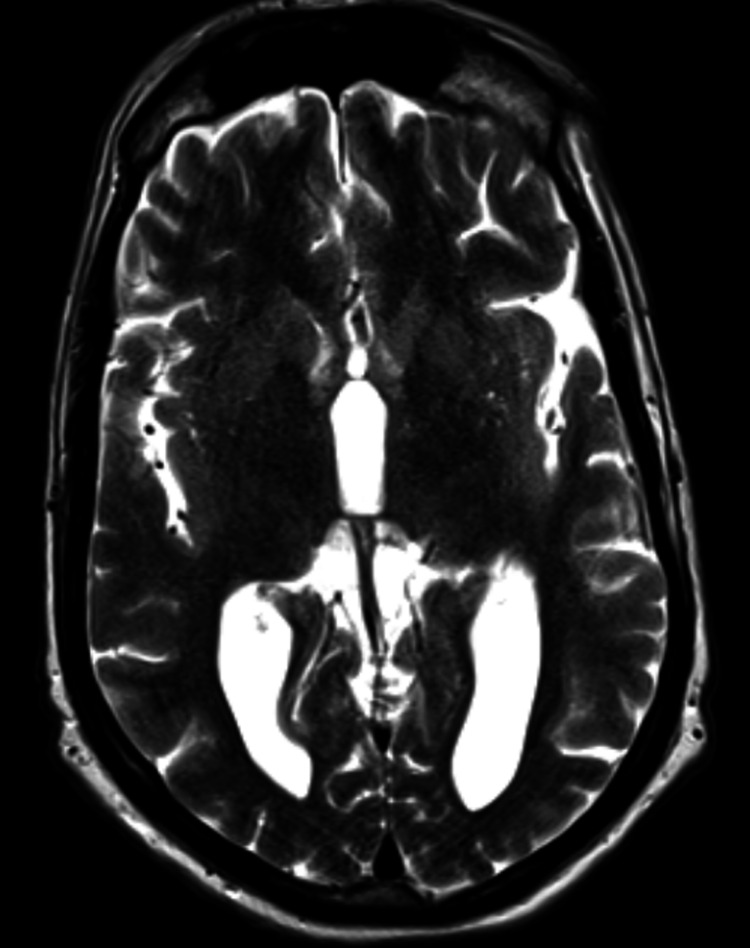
MRI of the brain without contrast (T2 sequence) negative for any mass, infarct, or demyelinating lesions
